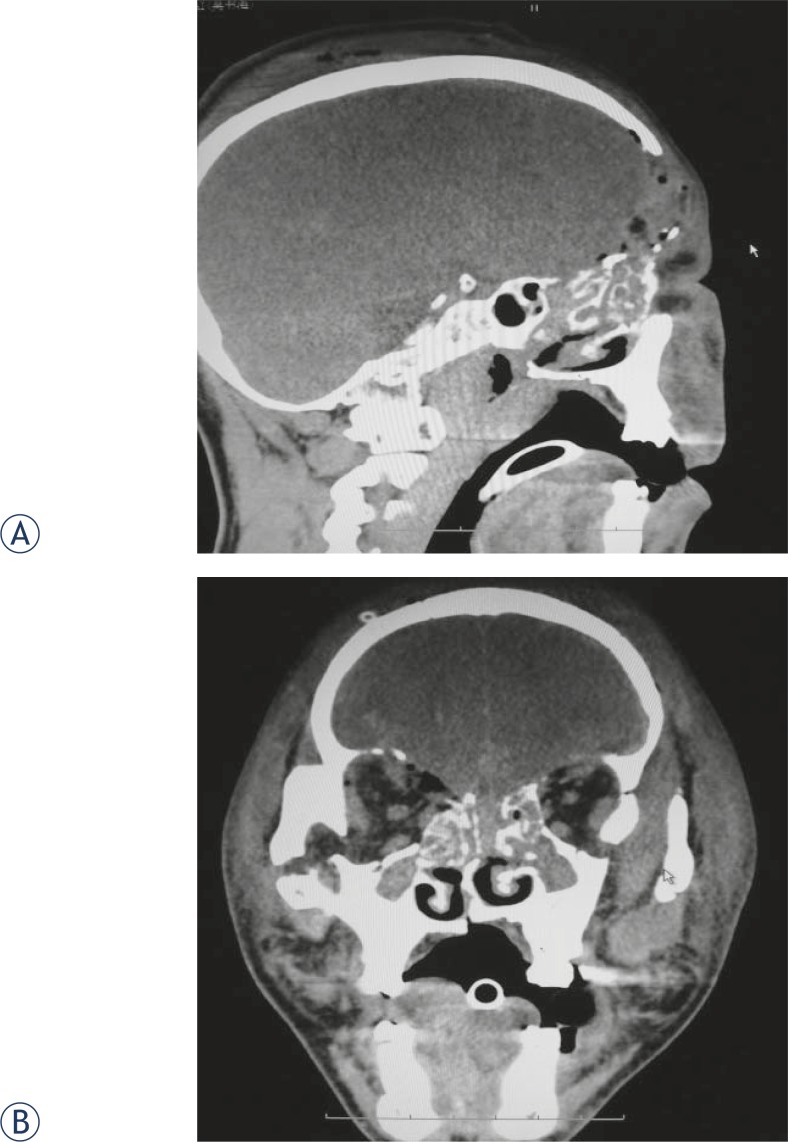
A 43-year-old patient diagnosed with multiple basilar skull fracture induced by severe craniocerebral injury. Partial frontal bone and superficial arch were resected and cerebrospinal rhinorrhea was present 10 days after surgery (Right). CT showed multiple basilar skull fracture. The cerebrospinal leak location could not be determined because of the several defects in ethmoid and sphenoidal sinus. It was demonstrated by surgery that meninges defect was present at the site of ethmoid sinus (A, sagittal view; B, coronal view). Rhinorrhea disappeared after the surgery repair.
